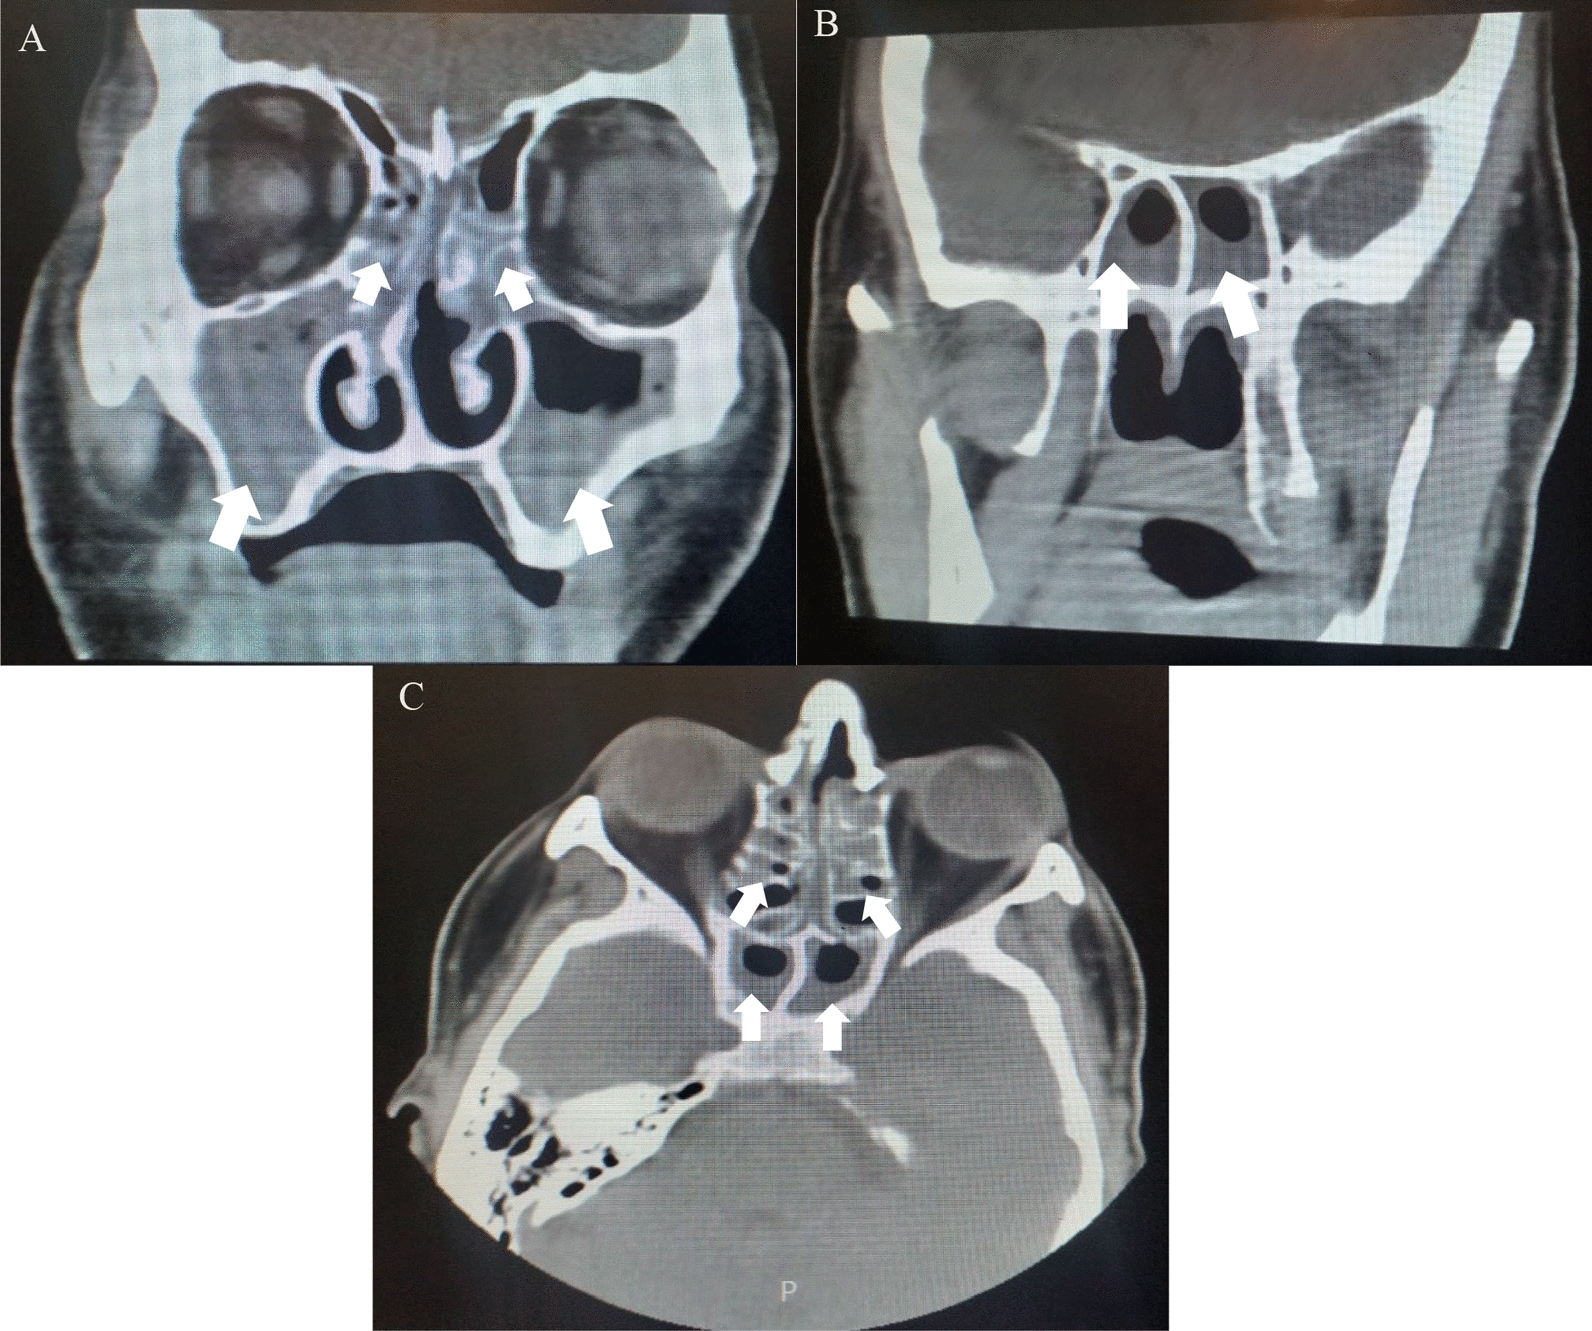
Paranasal sinuses computed tomography scan without contrast showing mucosal thickening of all paranasal sinuses. Note the fullness of all of the paranasal sinuses and their involvement as well as sinus edema (white arrows). A: coronal section of maxillary and ethmoid sinuses; B: coronal section of frontal sinuses; C: coronal section of paranasal sinuses showing ethmoid and sphenoid sinuses
